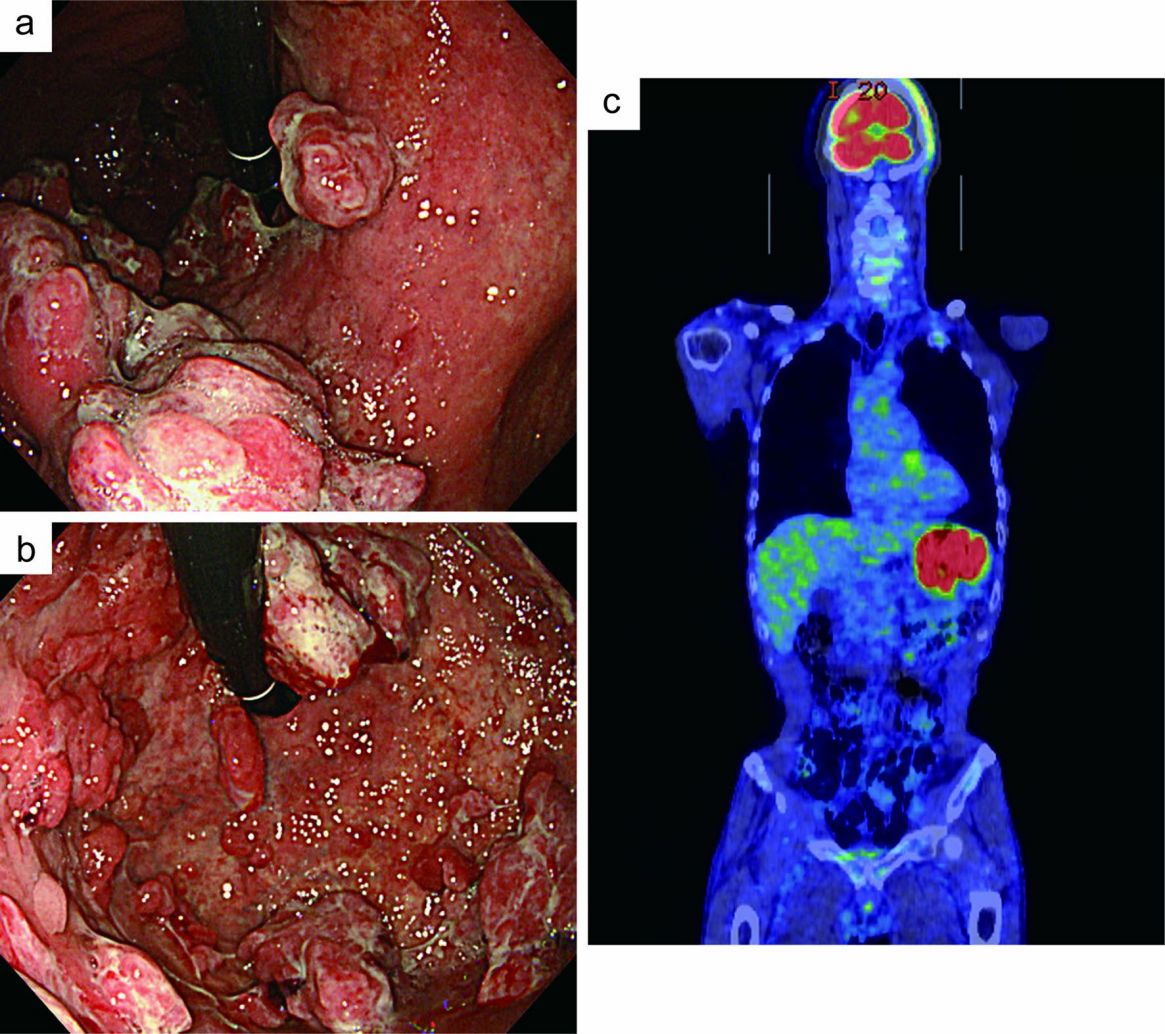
Endoscopic images and positron emission tomography/computed tomography images on initial examination. a Esophagogastroduodenoscopy showed multiple polypoid tumors in the body of the stomach. b Endoscopic image of the fundus of the stomach. c Positron emission tomography/computed tomography showed localized accumulation in the stomach
